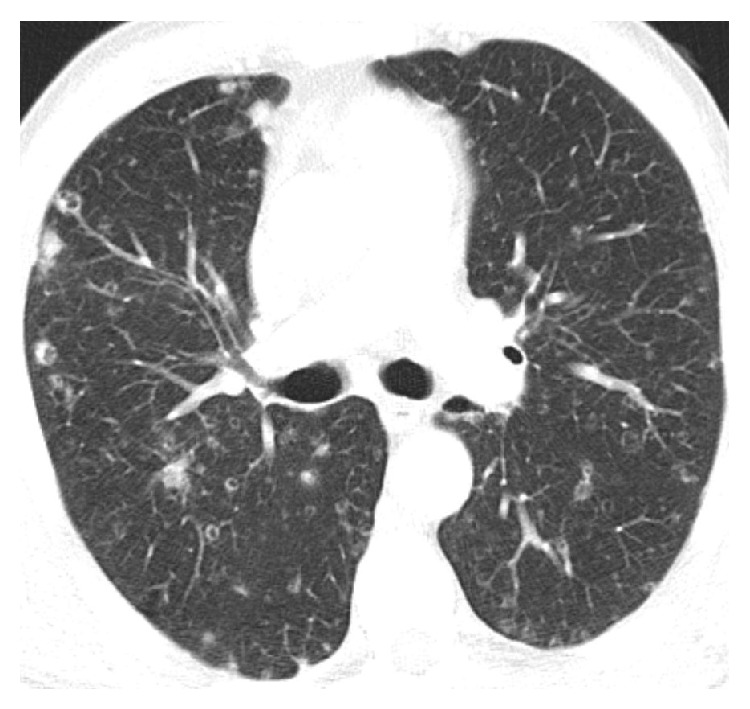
Axial thoracic CT image (lung window) in a lower thoracic CT slice: less nodules are identified. Note the normal appearance of the lung parenchyma other than the nodules.
